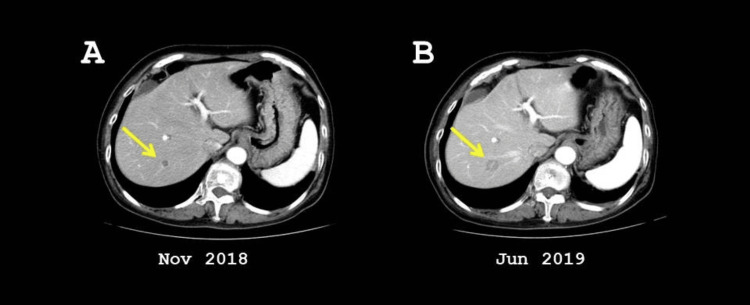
Abdominal CT images prior to RFA. (A) A hepatic metastasis in segment 7 from triple-negative breast cancer was identified in November 2018 (yellow arrow). (B) Despite chemotherapy with paclitaxel plus bevacizumab, the lesion increased in size by June 2019. CT: computed tomography; RFA: radiofrequency ablation.
